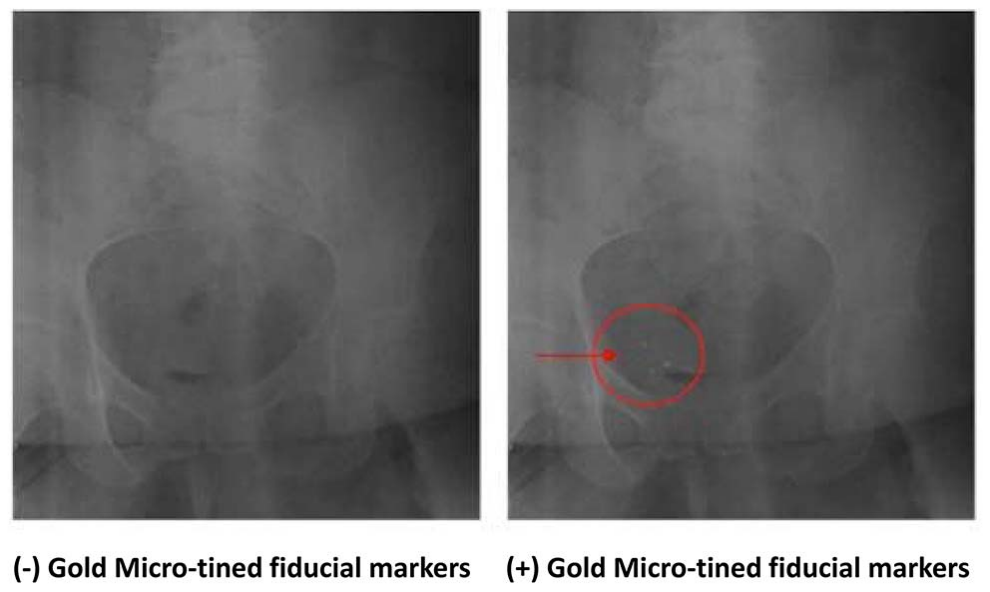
Plain-film X-Ray images of the pelvis *without* (left) and *with* (right) three 2.1×0.65 mm. 24K gold micro-tined fiducial markers surrounding the resection site of a right posterior-lateral bladder wall tumor.
